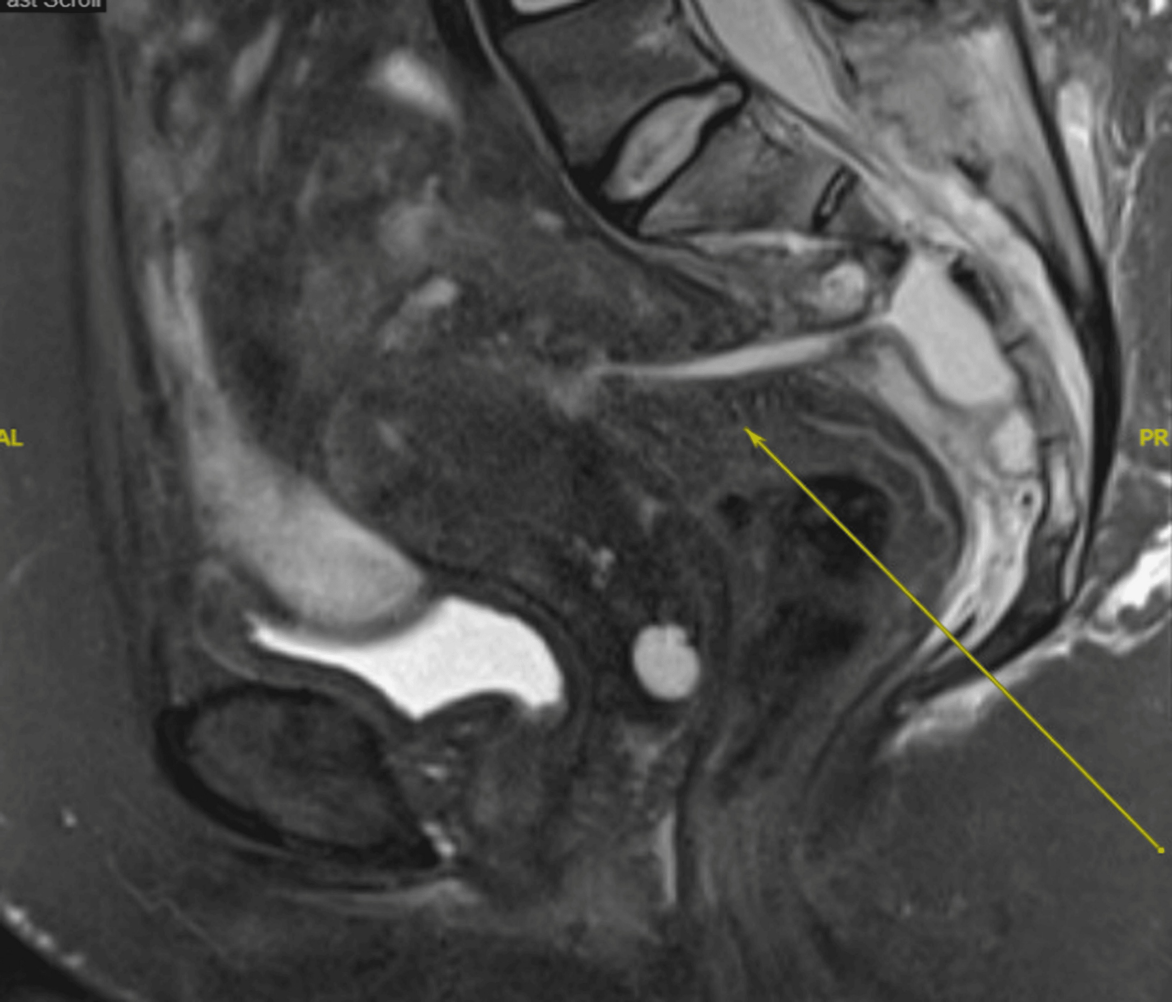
MRI with contrast: Fistulous tract extending from the superior medial aspect of the collection towards the fluid collection in the sacrum. The sacral collection extends posteriorly into the sacral spinal canal via an eroded defect in the sacrum, impressing on the thecal sac.
